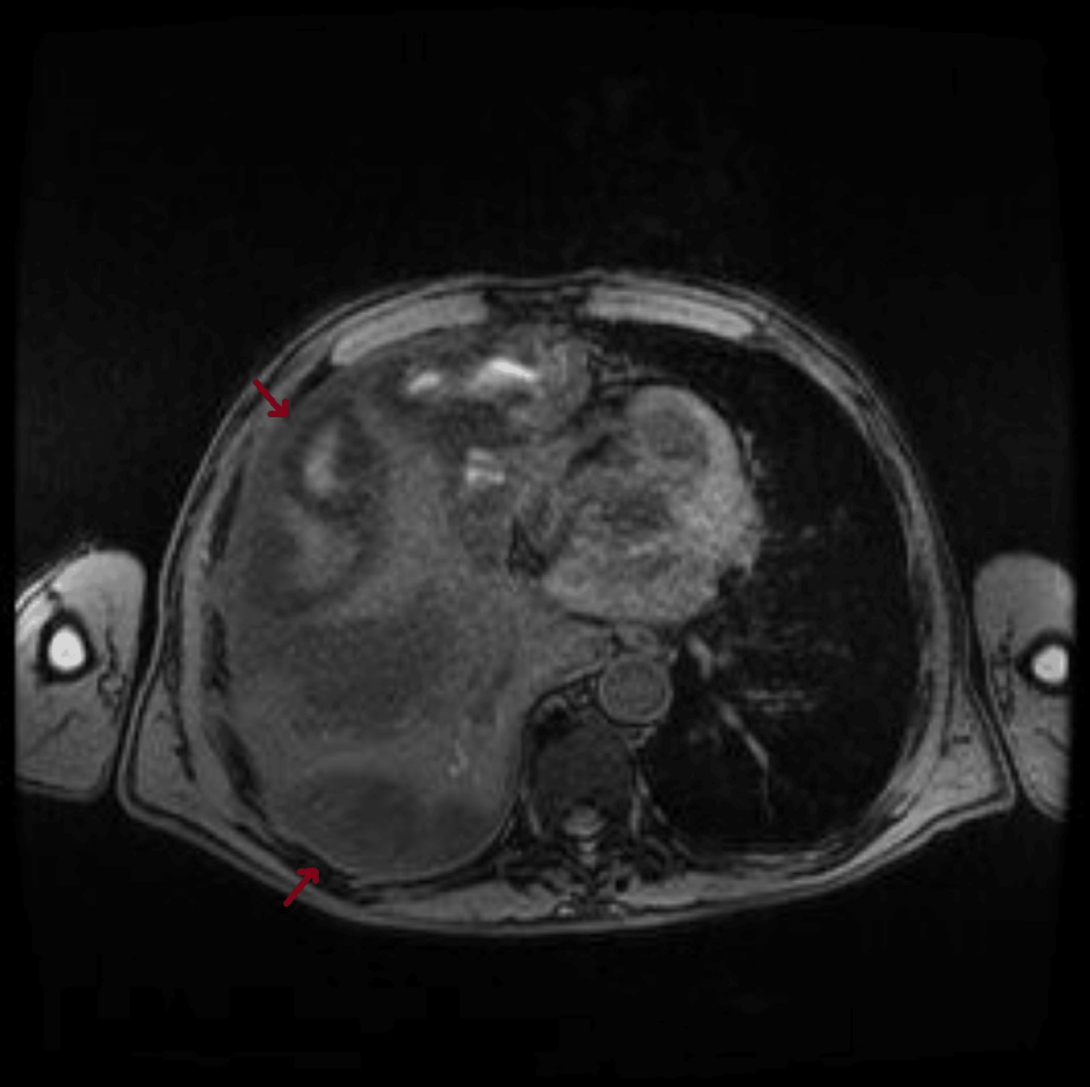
Abdominal MRI performed on the fourth day of hospitalization demonstrates numerous hepatic lesions (arrows), suggestive of hepatic abscesses, axial view.
